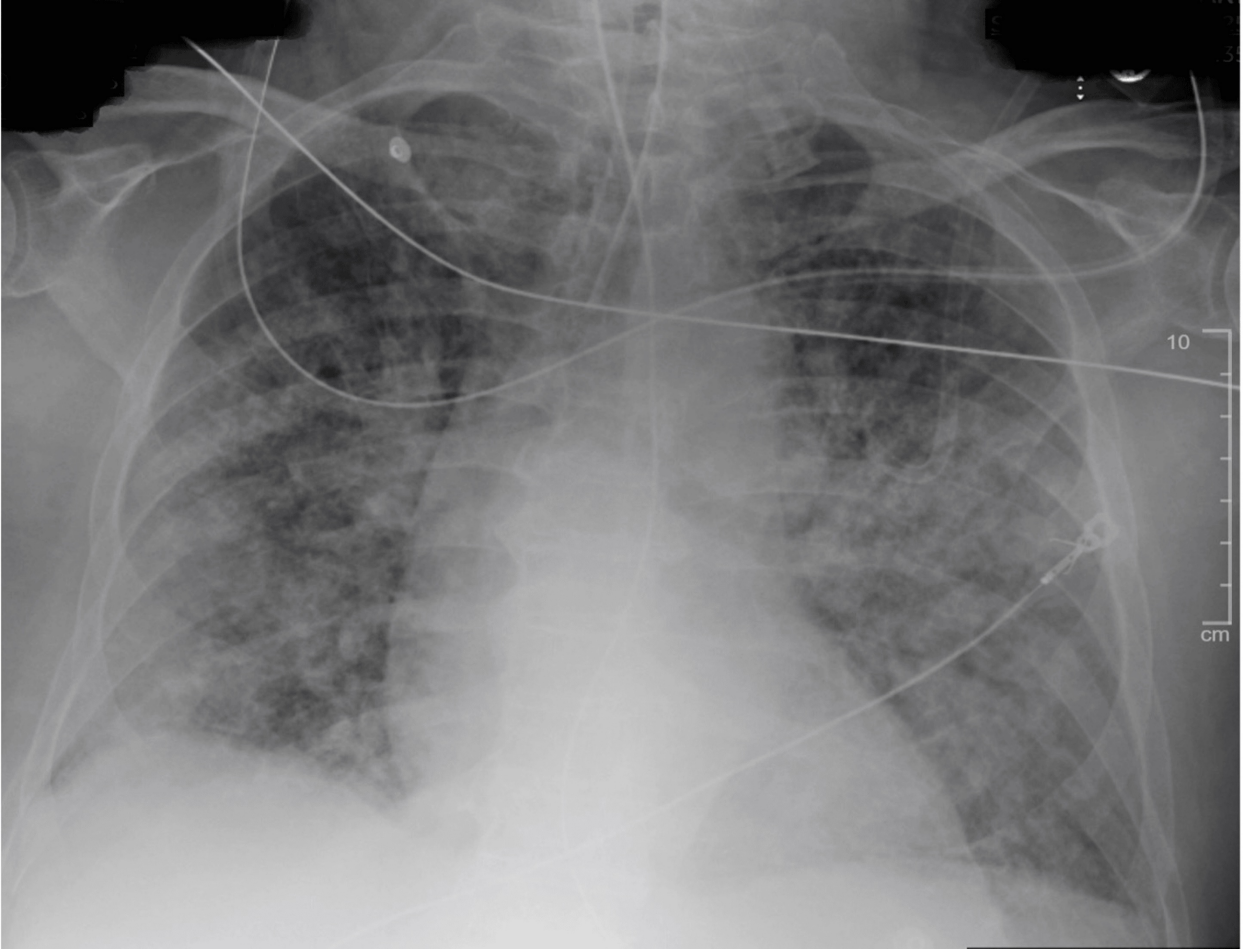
Chest X-ray on arrival to the emergency department. Patchy opacities are seen bilaterally.
